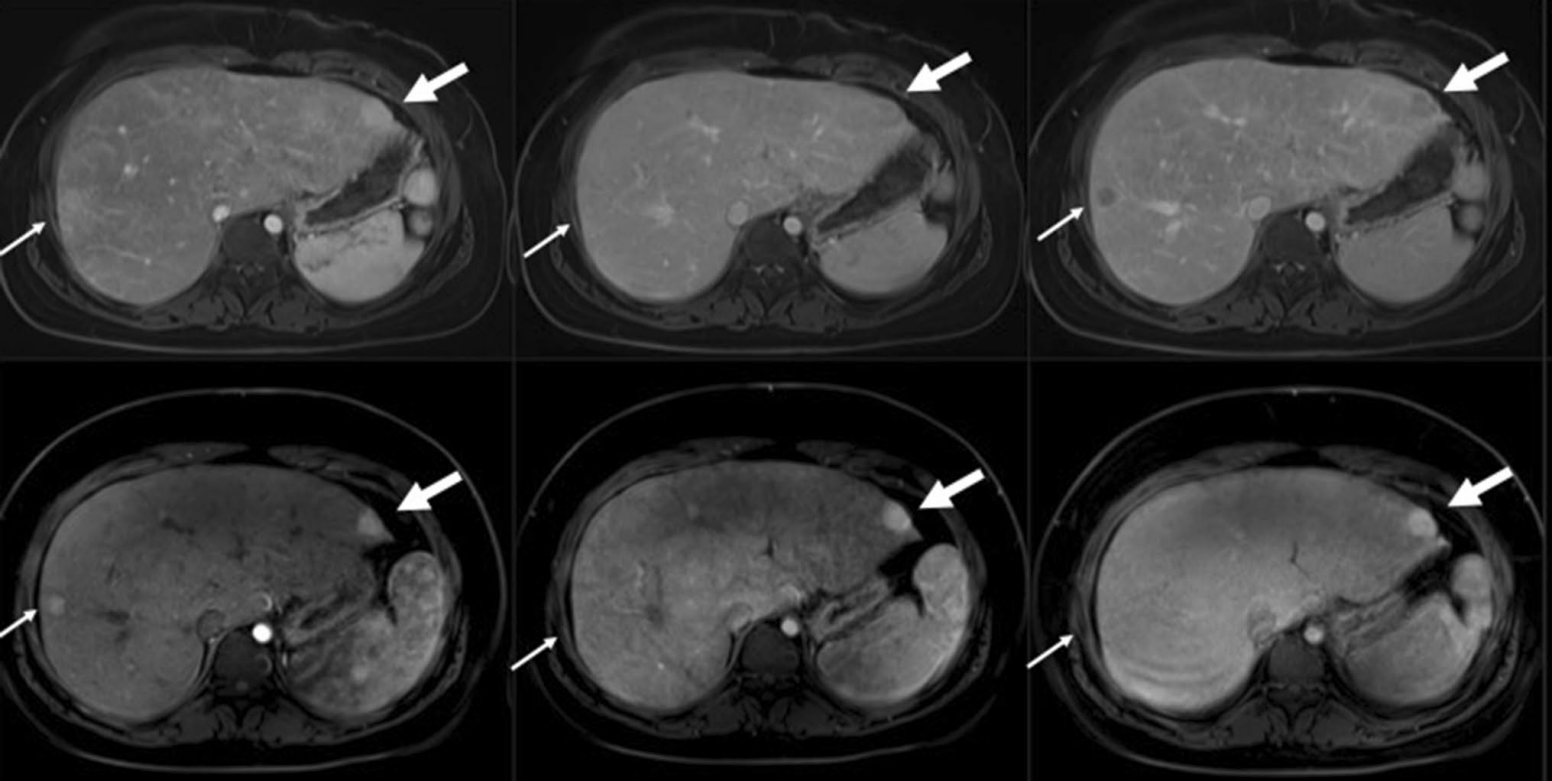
MRI of the liver of a 15-year-old girl with Fontan circulation and a morphologically left single ventricle. This is the same patient as in Fig. 4 showing contrast enhancement in two other nodules, one in the lateral part of the right liver lobe (thin arrows) and one in the left liver lobe (thick arrows). The upper row represents the initial examination with gadoterate meglumine and the lower row the first follow-up examination with gadoxetic acid. Images from left to right are in late arterial*/*arterial phase, portal venous phase and delayed portal venous phase. The initial examination reveals arterial enhancement of the two nodules with an isointense signal in the portal venous phase and a clear ‘wash-out’ in the delayed portal venous phase. In the follow-up examination, the portal venous and delayed phases display a homogeneous contrast enhancement of the nodules
